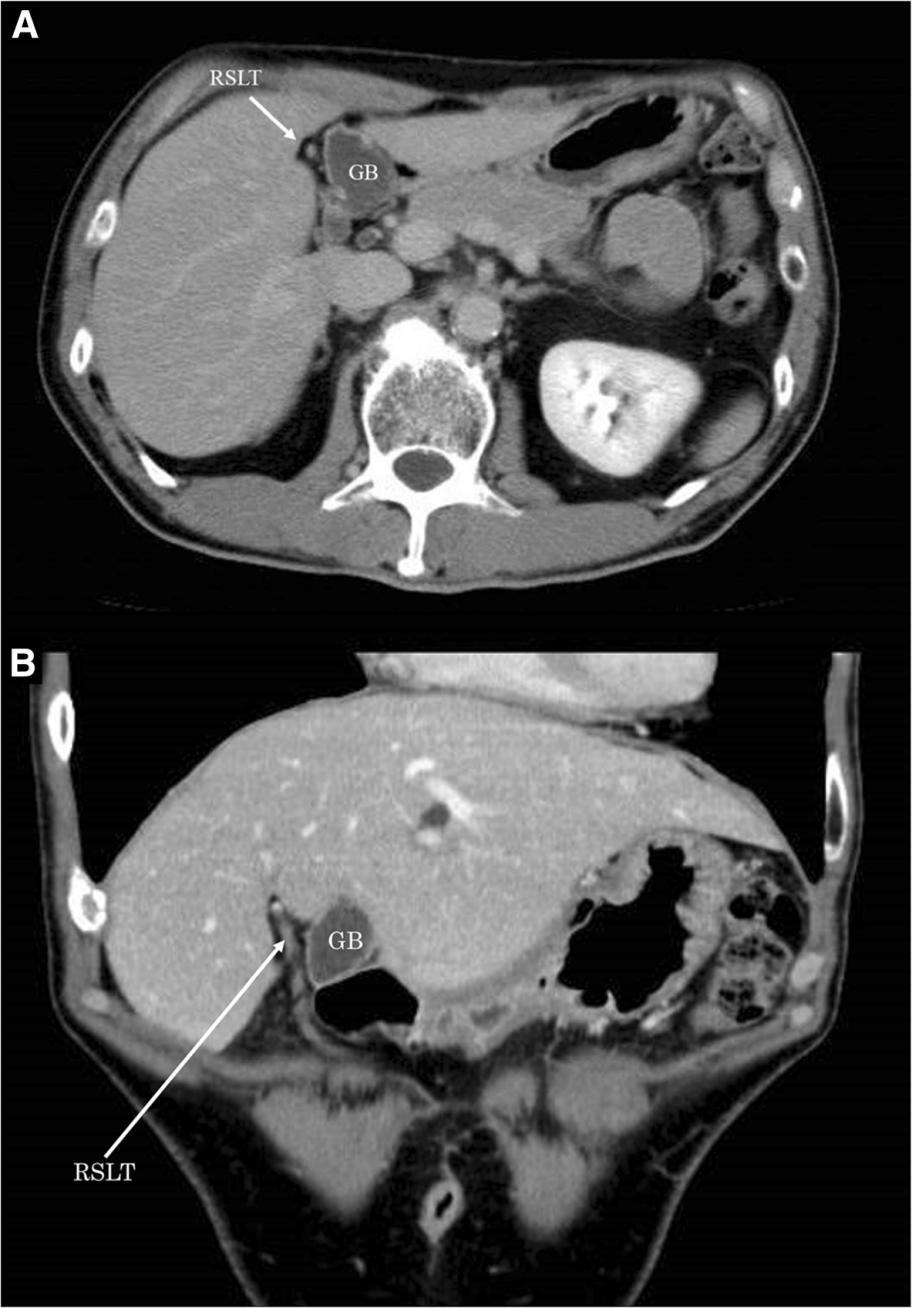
a Axial contrast-enhanced CT showed the ligamentum teres observed on the right side of the gallbladder (arrow). GB, gallbladder; RSLT, right-sided ligamentum teres. b Coronal contrast-enhanced CT showed the ligamentum teres observed on the right side of the gallbladder. GB, gallbladder; RSLT, right-sided ligamentum teres
